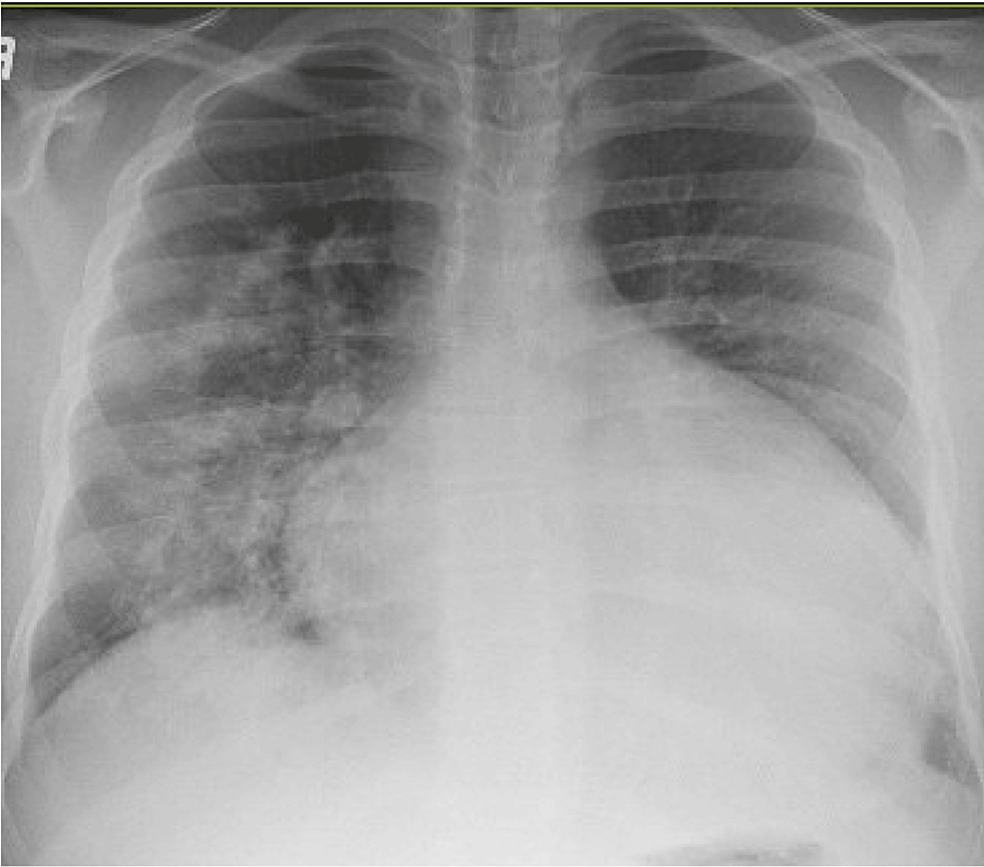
Chest X-Ray on admission Chest X-ray on admission showed prominent cardiomegaly and pulmonary congestion
